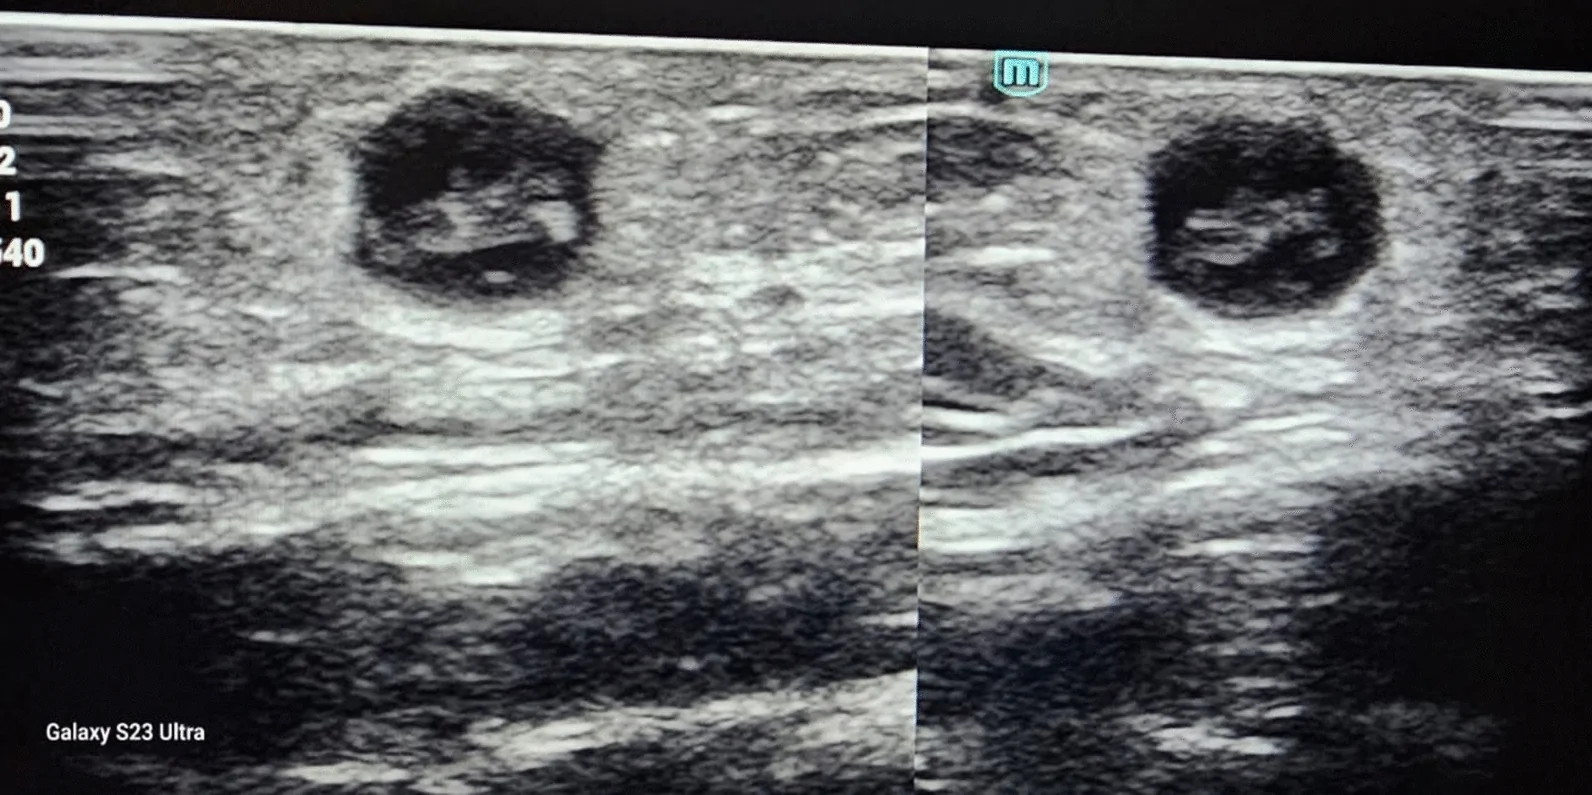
Scrotal ultrasonography demonstrating mobile echogenic structures within dilated lymphatic channels—the characteristic “filarial dance sign” indicating live adult filarial worms in the lymphatic vessels
